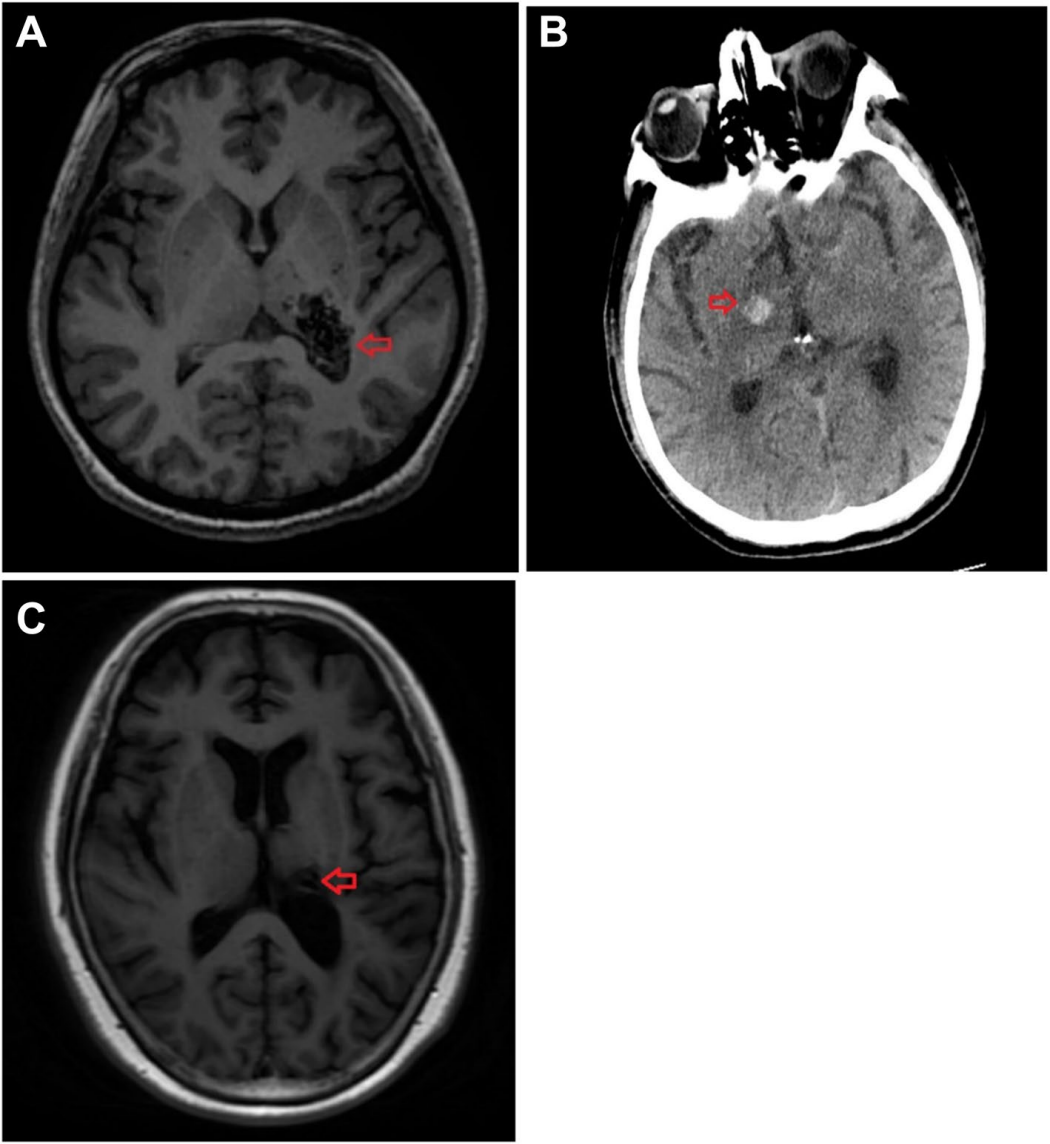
Cranial imaging of the three patients. A MRI of patient #1; B CT of patient #3; C MRI of patient #2
